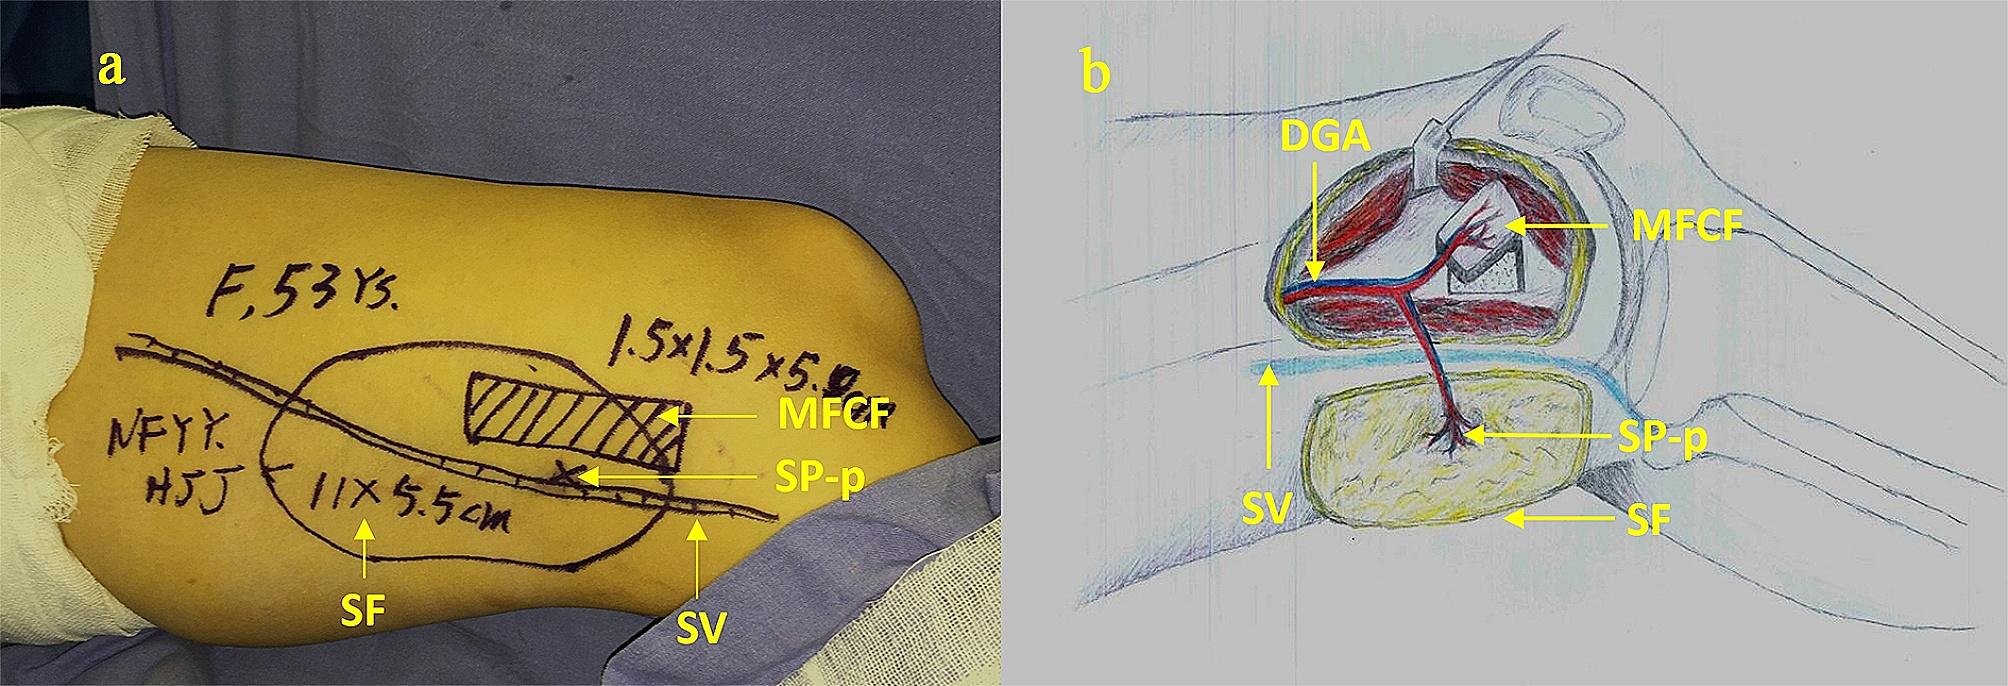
After the use of preoperative CTA and intraoperative Doppler ultrasound, the initial marking of the incision line in case 2 (3a). The entire chimeric DGAP flap (MFCF and SF) was completely isolated, and the SV was preserved (3b)
